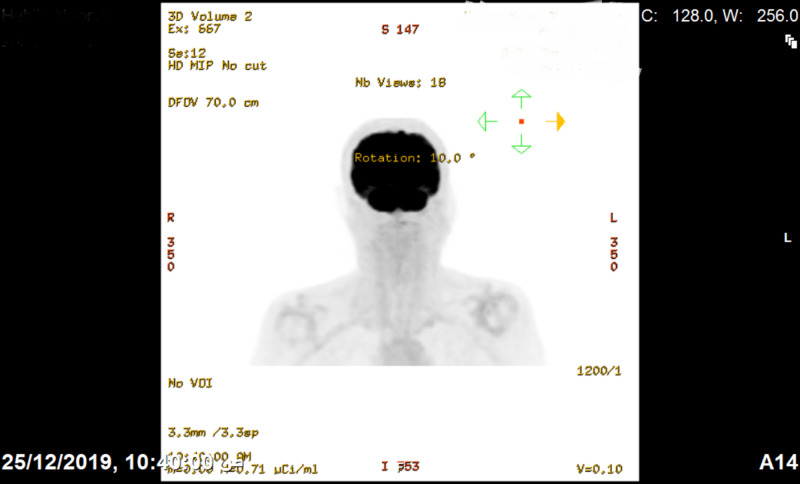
FDG-PET scan revealed no dominant FDG uptake in the nasopharyngeal region. FDG-PET: positron emission tomography with fluorodeoxyglucose
